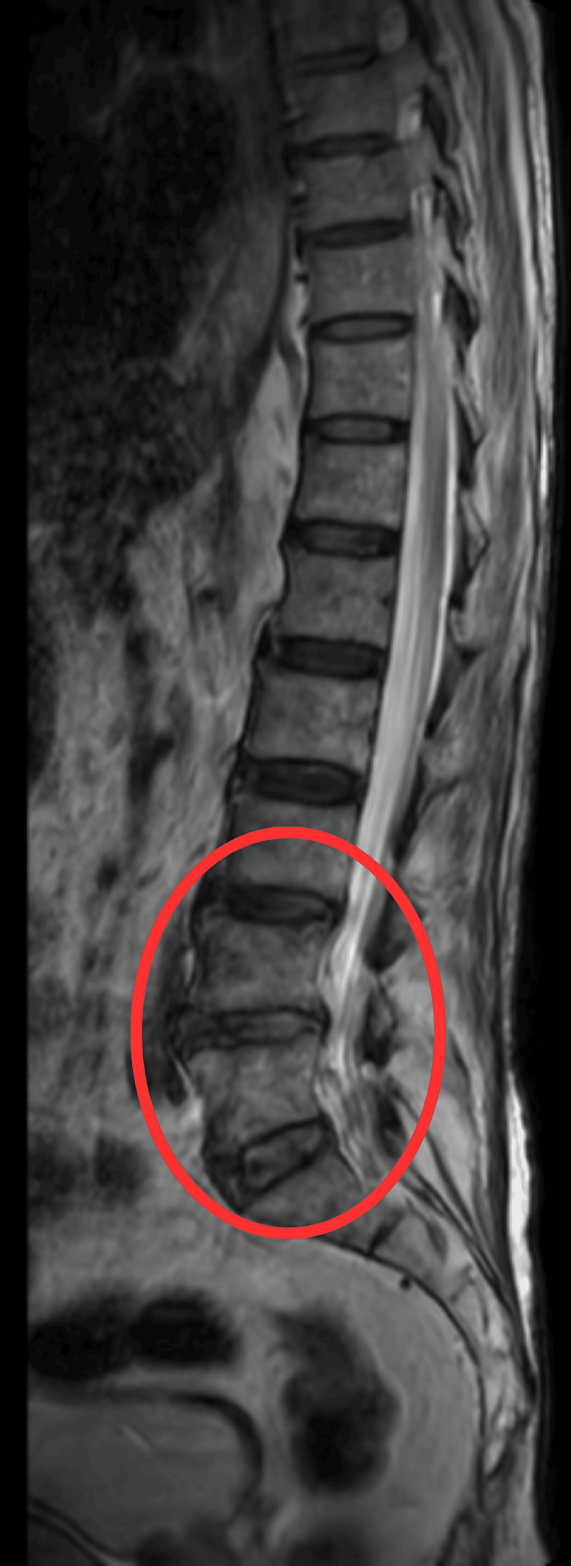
MRI of the whole spine The circle shows L2-3 to L5-S1 level mild posterior disc bulge seen indenting anterior thecal sac, and no central canal stenosis MRI: magnetic resonance imaging
